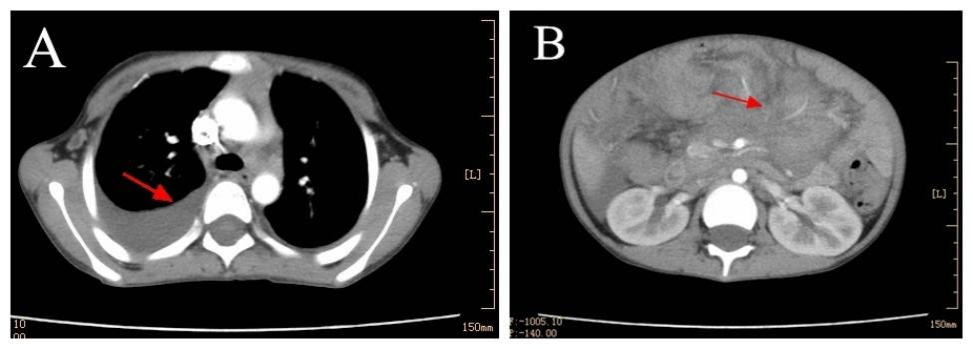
Thoracoabdominal enhanced CT. (A) Bilateral pulmonary inflammation, pleural effusion, and pleural thickening, along with enlarged lymph nodes in the mediastinum. (B) Thickening and swelling of the intestinal wall in the abdominal cavity
